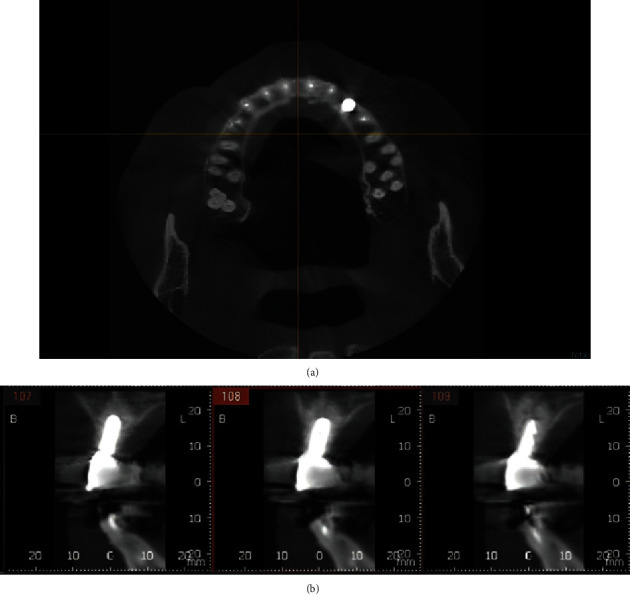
CBCT after 12 months. (a) Occlusal view. (b) Sagittal views.
